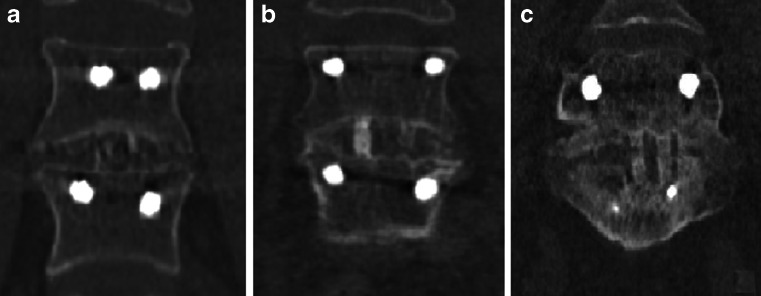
Examples of CT fusion scores 0 (a), 1 (b) and 2 (c)
